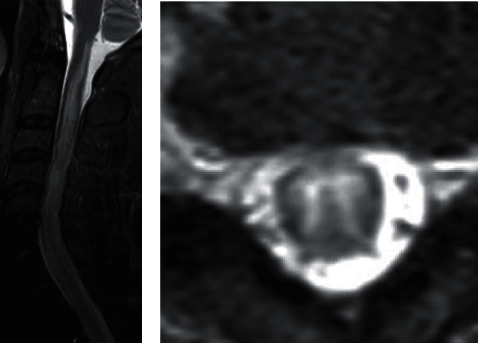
(a) MRI cervical spine sagittal STIR. Longitudinally extensive T2 hyperintense lesion with cord expansion from the cervicomedullary junction to C7. (b) MRI cervical spine axial T2. Prominent grey matter T2 hyperintensity.
